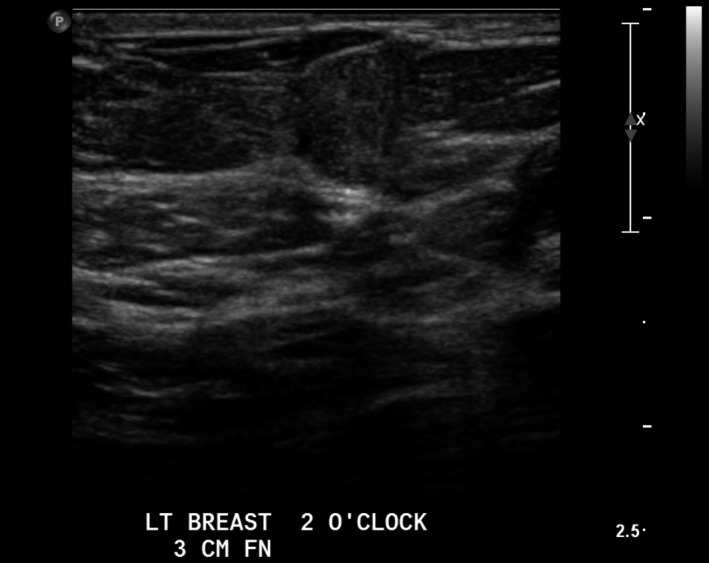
Lesion two: Ultrasound image showing a superficial, round mass adjacent to Lesion 1. Image courtesy of BreastScreen ACT.
